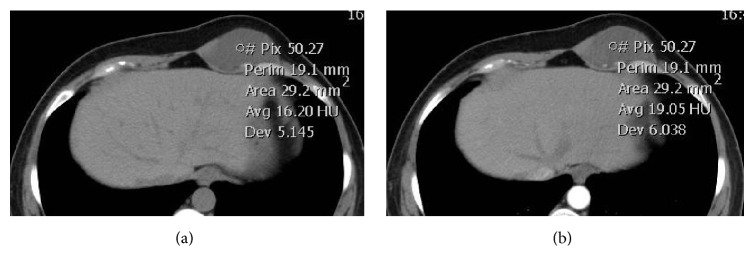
Typical radiological appearance from a 48-year-old man with EMC of the walls of the chest. Transverse CT scan showed low density, irregular mass ((a), CT value=16.2Hu) without marked enhancement ((b), CT value=19Hu in CT Contrast Enhancement).
